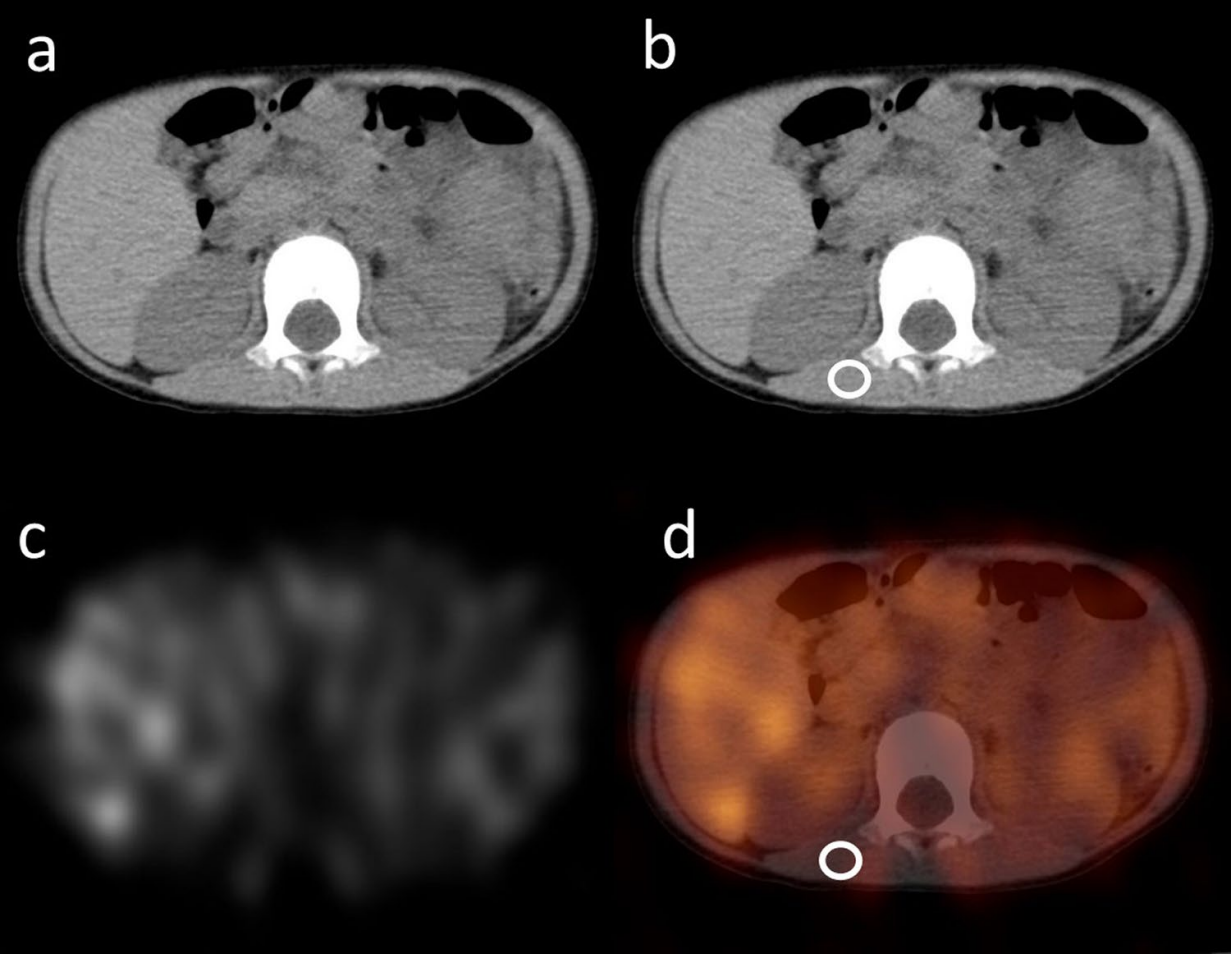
CT (a, b), SPECT (c) and SPECT/CT fusion image (d) of case No. 4. For the VOI of muscle in this case, 1-cm-diameter sphere of VOI was settled in the second vertebrae level of the right latissimus dorsi muscle (b, d; circled area)
